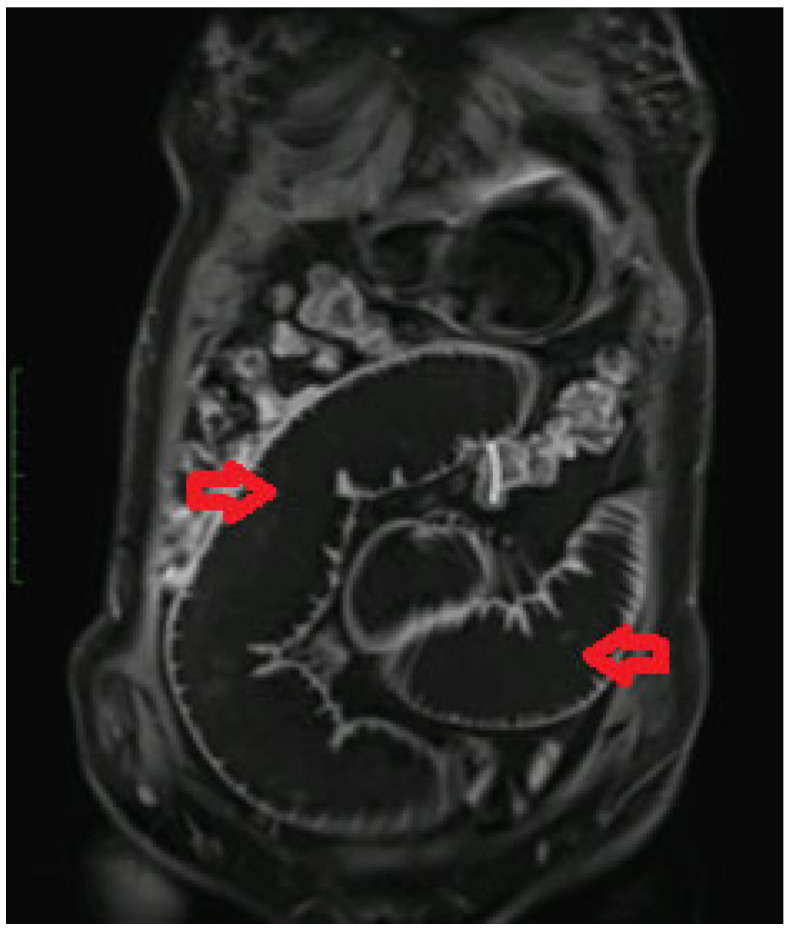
Entero-MRI which revealed a high intestinal obstruction with multiple distended jejunal loops visible marked by red arrows.
